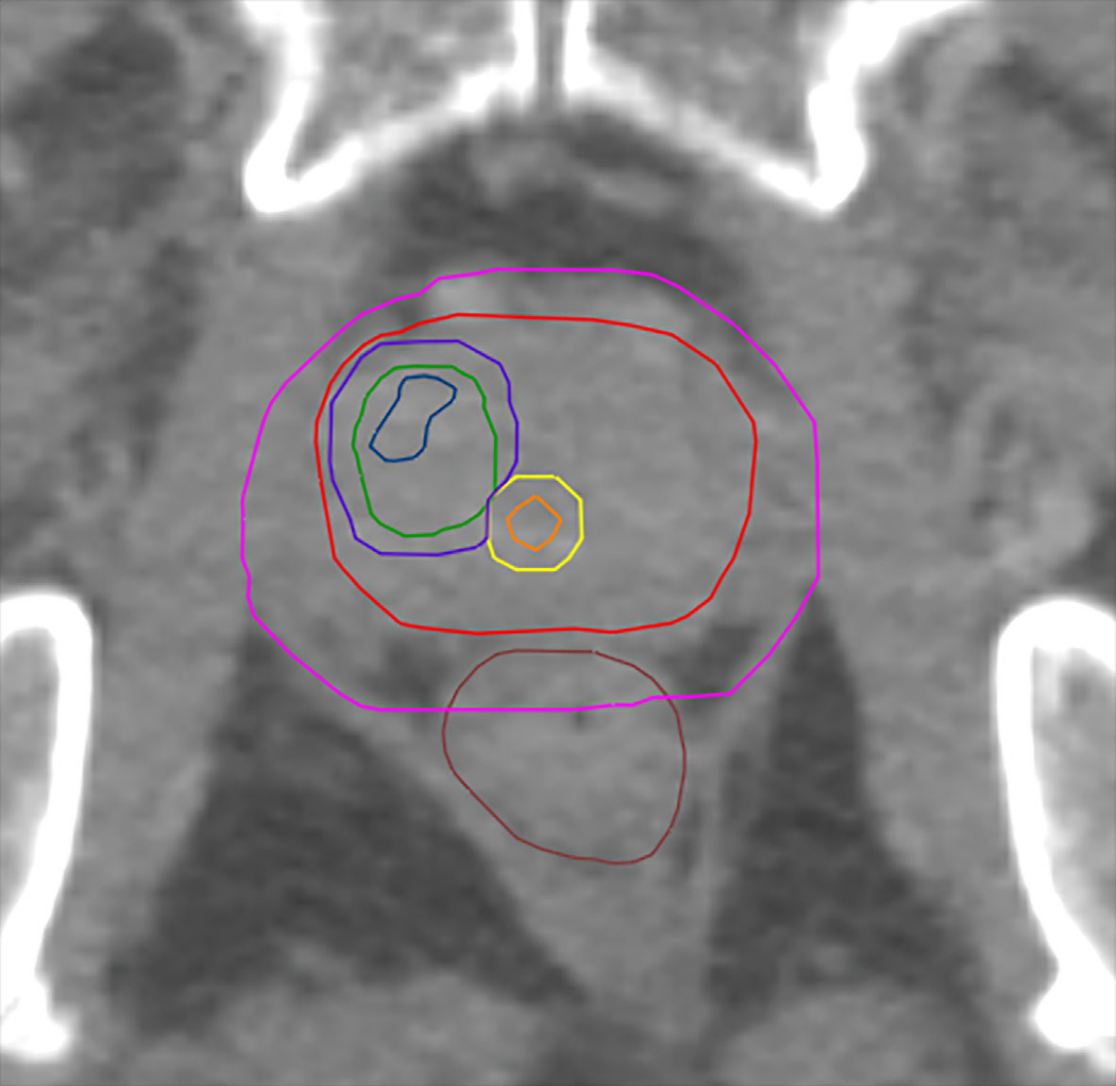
Shows GTV-MRI (blue), GTV-PET (green), urethra (orange) PRV-urethra (yellow), prostate (red), PTV3_3 (boost volume minus PRV-urethra, purple) and PTV1 (pink).
